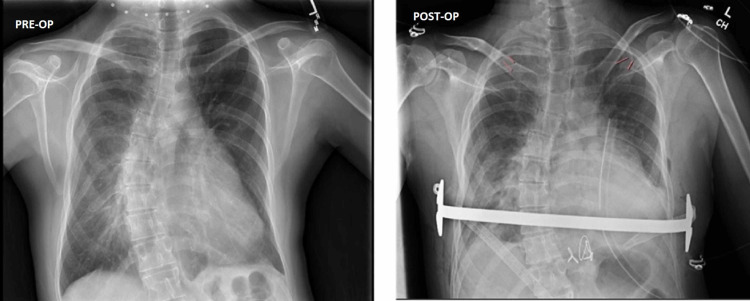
Preoperative (left) and postoperative (right) chest radiographs
